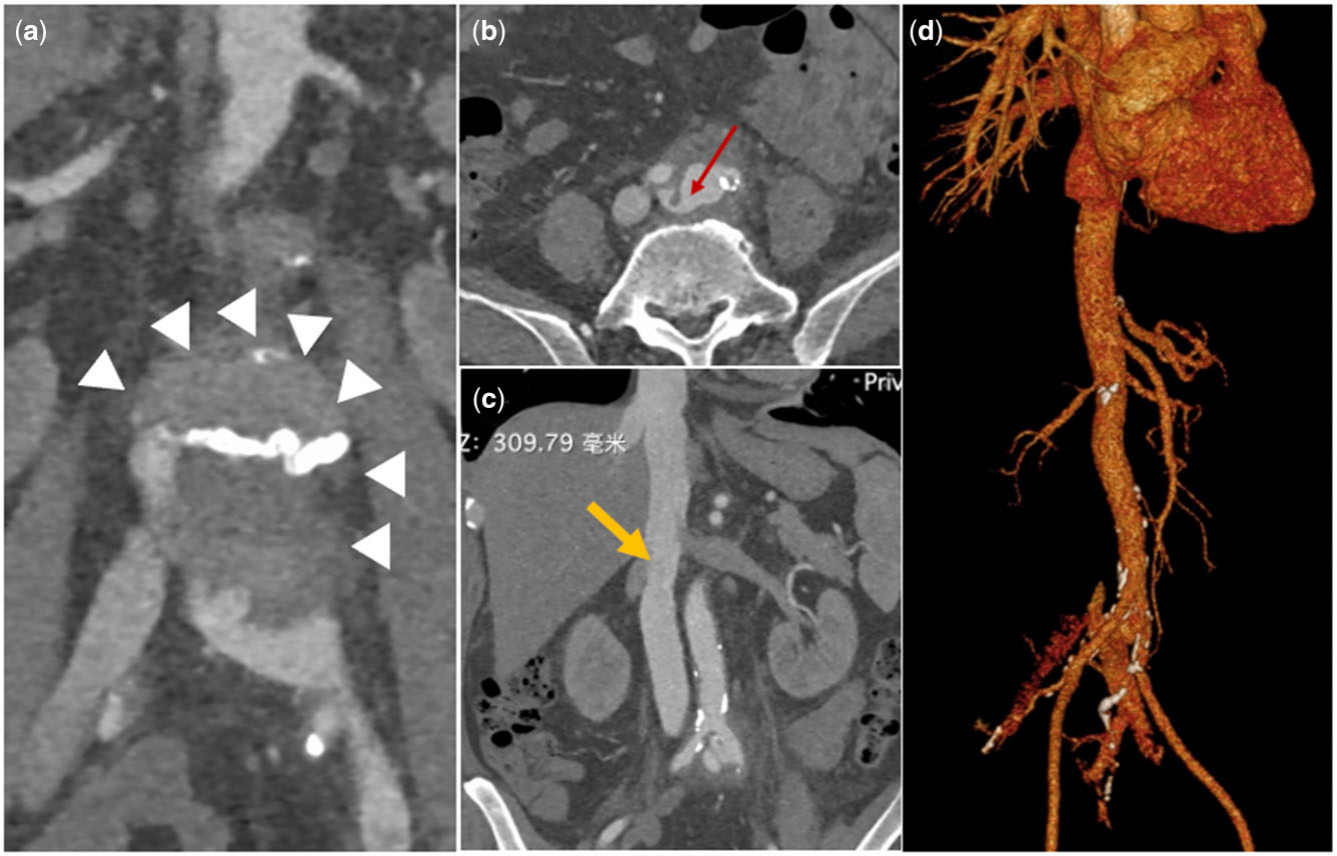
Computed tomography angiography (CTA) shows aortic pseudoaneurysm (a, arrowhead) with arterio-venous fistula (b, arrow). Inferior vena cava (c, arrow) in the coronal view of CTA. The fistula between the right iliac artery and left iliac vein (d).
